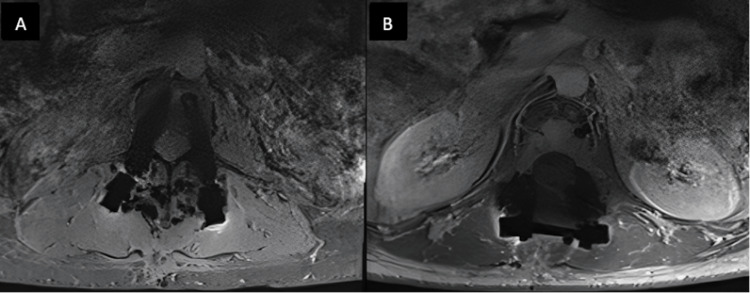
Postoperative magnetic resonance imaging scan obtained prior to stereotactic radiotherapy. T1-weighted images with intravenous gadolinium contrast. A: axial cut at the level of T12. Note carbon-fibre screws with minimal artefacts and good visibility of the content of the spinal canal. B: axial cut at the level of L1 with enhancing postoperative changes within the resection cavity and no compression of the theca, facilitating safe delivery of stereotactic radiotherapy.
